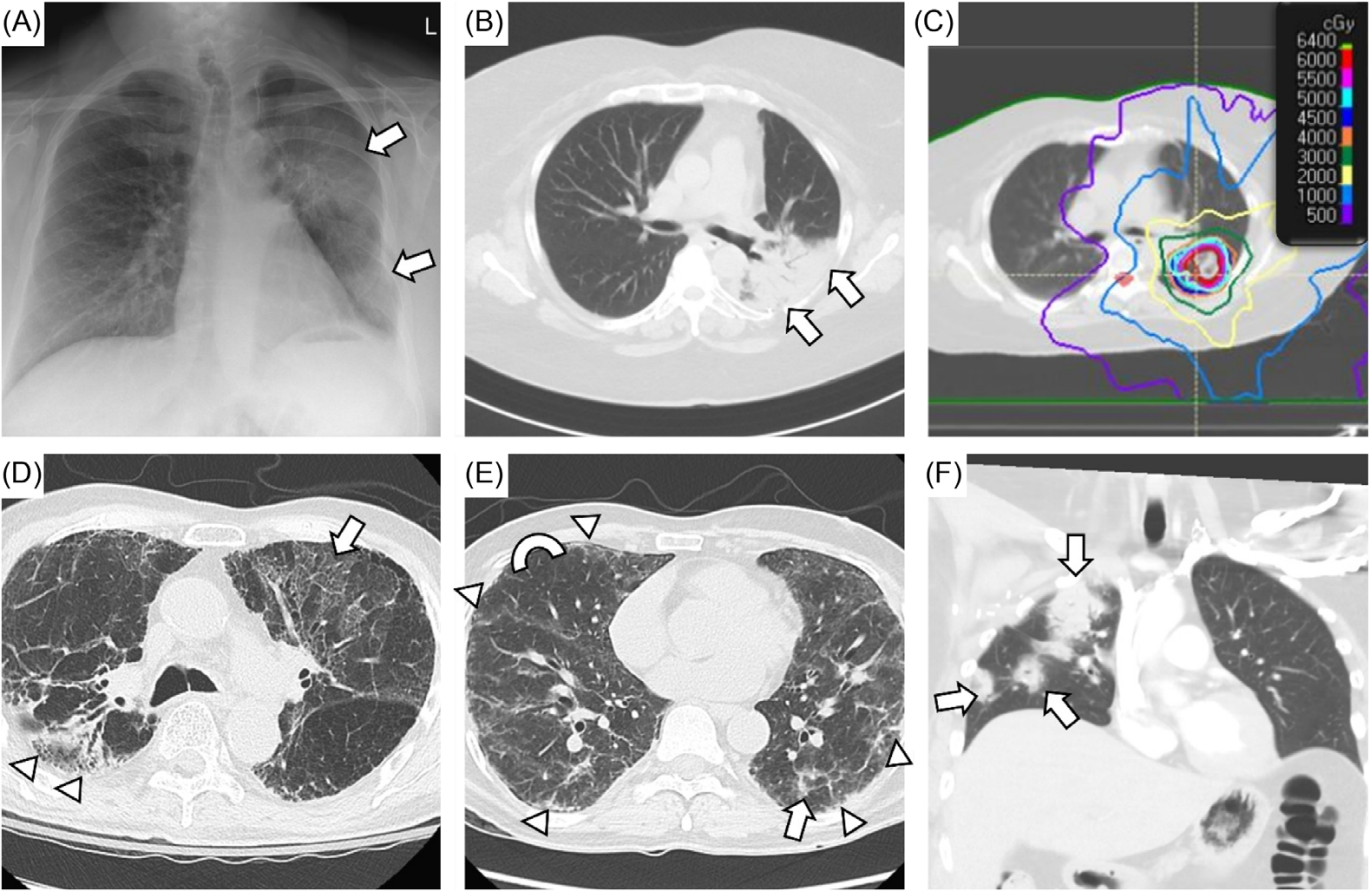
Challenging cases of possible immune checkpoint inhibitor (ICI) pneumonitis. A-C) Middle-aged woman with metastatic renal cell carcinoma treated with cabozatinib and nivolumab and radiation to the left upper lobe. She presented with cough and dyspnea with exertion (Grade 2) six months after radiation therapy was completed. Chest radiograph reveals new infiltrates in the left lung (arrows), and computed tomography of chest (CT chest) demonstrates dense consolidation in the left upper lobe with air bronchograms (B, arrows). Her infiltrates corresponded to radiation field (C). She was diagnosed with radiation-related lung injury and improved with oral steroid; however, ICI-associated pneumonitis could have also contributed. D) Elderly man with non-small cell lung cancer who underwent definitive chemoradiation therapy to the right upper lobe followed by pembrolizumab. He was hospitalized after a fall, and he was noted to have low oxygen saturations. CT chest revealed post-treatment changes in the right upper lobe (arrowheads) and bilateral ground-glass upper lobe infiltrates (arrow) in the setting of diffuse emphysematous changes. He was treated for chronic obstructive pulmonary disease (COPD) exacerbation with empiric antimicrobial therapy and oral steroids. His steroids were prolonged given lack of clinical improvement, so ICI-associated pneumonitis was also a concern. Bronchoscopy was not feasible due to significant oxygen requirement. E) Middle-aged man with papillary thyroid cancer who had undergone resection and treatment with carboplatin and paclitaxel. He was subsequently treated with pembrolizumab and presented with cough and dyspnea with exertion. He also had history of vocal cord dysfunction with paralyzed left vocal cord and aspiration risk. CT chest revealed diffuse peripheral and subpleural thickening (arrowheads), ground-glass opacities (arrow), and mosaic attenuation (semicircle showing contrast). He underwent bronchoscopy with lavage, and he was treated empirically for infection and with IV steroids for possible drug-related pneumonitis. Other potential etiologies included aspiration pneumonia, interstitial lung disease, and COPD exacerbation. F) Middle-aged woman with HER2-positive breast cancer treated with pembrolizumab hospitalized for fever, cough and dyspnea with exertion. Coronal CT chest reveals patchy opacities (arrows) affecting the right upper, middle, and lower lobes. Bronchoscopy was performed, and no obvious infection was found. She was treated with empiric antimicrobial therapy and iIV steroids for presumed ICI-associated pneumonitis. She was discharged on oral steroids therapy with *Pneumocystis jirovecii* prophylaxis.
